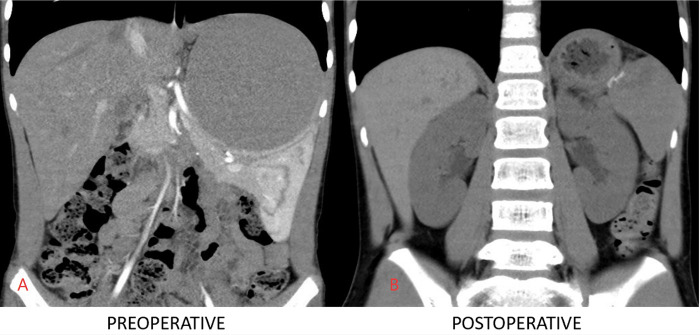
Preoperative and postoperative computed tomography findings. (A) Preoperative coronal computed tomography image showing a giant splenic cystic lesion with marked mass effect on adjacent structures. (B) Postoperative coronal computed tomography image demonstrating satisfactory residual splenic morphology without evidence of cyst recurrence.
